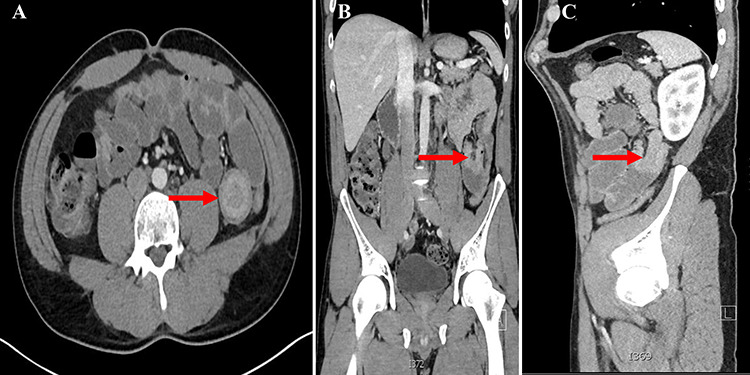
A. CT abdomen axial view showing the ‘Target Sign’ present within the small bowel; B. CT abdomen coronal view showing invagination of a proximal portion of small bowel into the adjacent section of bowel; C. CT abdomen sagittal view showing the aforementioned invagination of small bowel.
